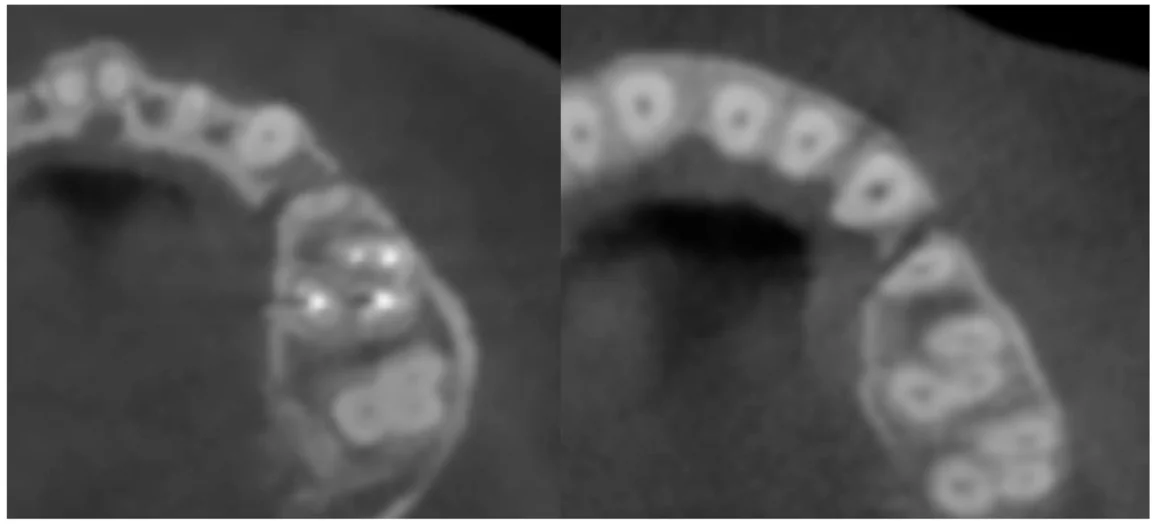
CBCT image showing minor dental injury.
